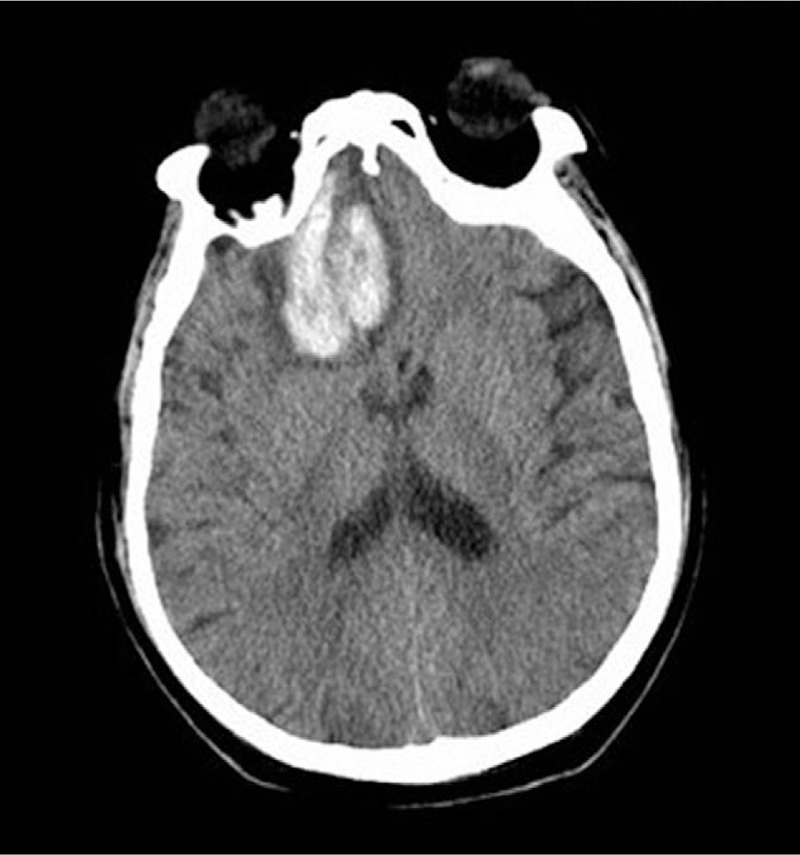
Cerebral CT revealed hematoma in the area of right frontal lobe.
